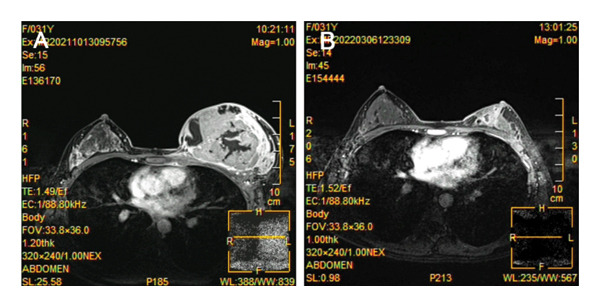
MRI contrast before and after neoadjuvant therapy. (A) Before neoadjuvant therapy. (B) After neoadjuvant therapy in 8 cycles.
